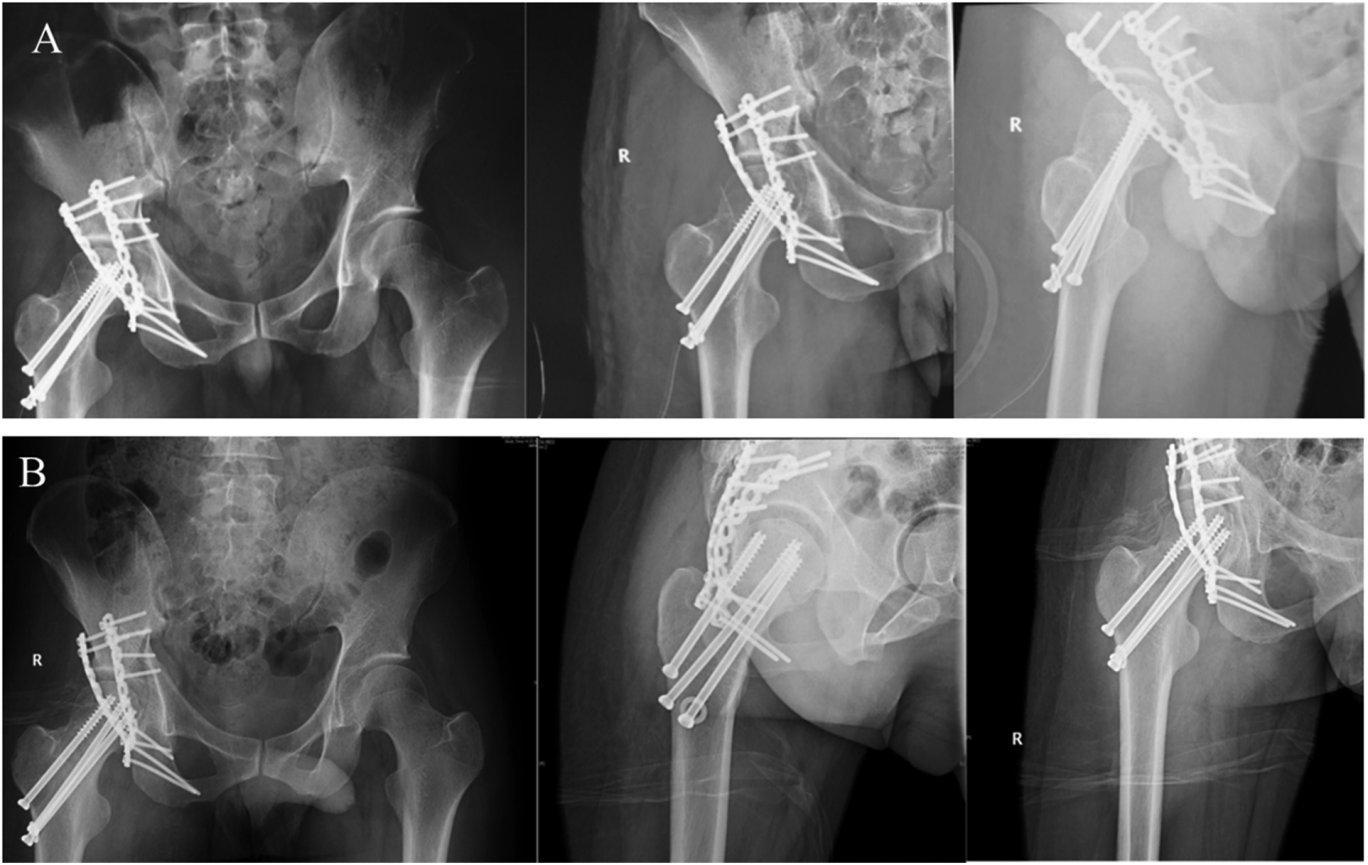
The postoperative review and 1 month follow-up of hip fracture triad. (A) The patient was placed under general anesthesia in the left lateral position. The K-L approach of the right hip was taken to separate and expose the femoral neck fractures, posterior hip dislocation and posterior wall fractures of the acetabulum layer by layer. The femoral neck fracture was first reduced and the hip joint was fixed with three hollow screws. The posterior wall and posterior column fractures of the acetabulum were fixed with two reconstruction plates. (B) Radiographs of pelvis, ilium obliquity and obliquity of obliquity 1 month after operation showed the general structure of hip joint.
